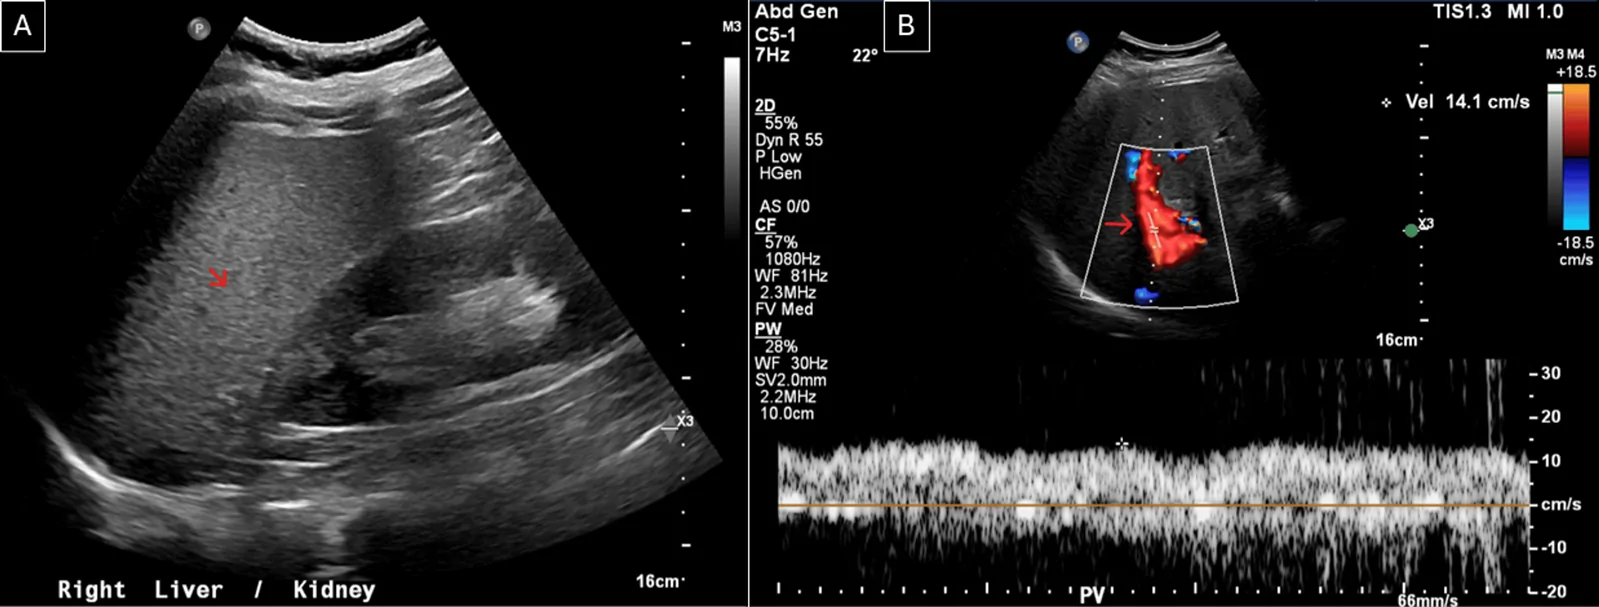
Abdominal ultrasonography demonstrating chronic hepatic steatosis with patent hepatic vasculature. (A) Representative ultrasound image of the right hepatic lobe demonstrating diffusely increased hepatic echogenicity (red arrow), consistent with hepatic steatosis. (B) Color Doppler ultrasound demonstrating a patent main portal vein (red arrow) with preserved hepatopetal flow.
